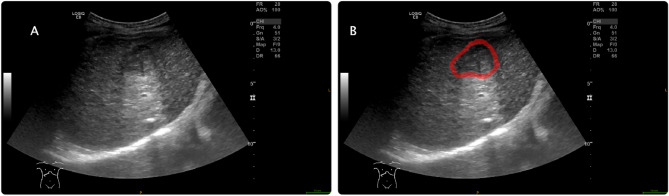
Example of region of interest delineation on ultrasound imaging using ITK-SNAP software. (A) The original ultrasound image of the liver lesion. (B) The same ultrasound image with ROI outlined in red, demarcating the area around the lesion for subsequent radiomic analysis. ROI, region of interest.
